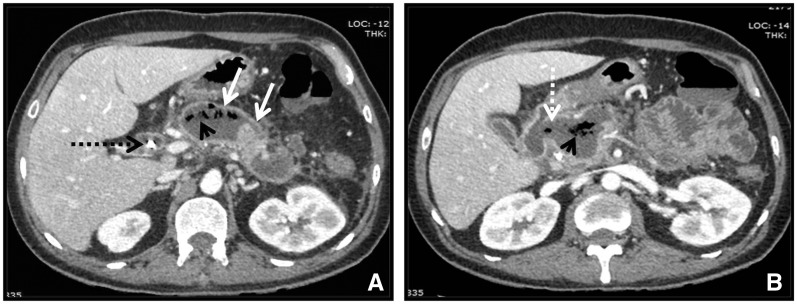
Images from a 44-year-old male 6 weeks after an episode of necrotizing pancreatitis with walled-off necrosis (WON). (A) Axial contrast-enhanced CT showing thick enhancing wall (arrows) around a walled-off necrotic collection with air foci (arrowheads). Also note dilated common bile duct with stent in situ (black dashed arrow). (B) Caudal image showing communication of WON with medial wall of duodenum (white dashed arrow).
